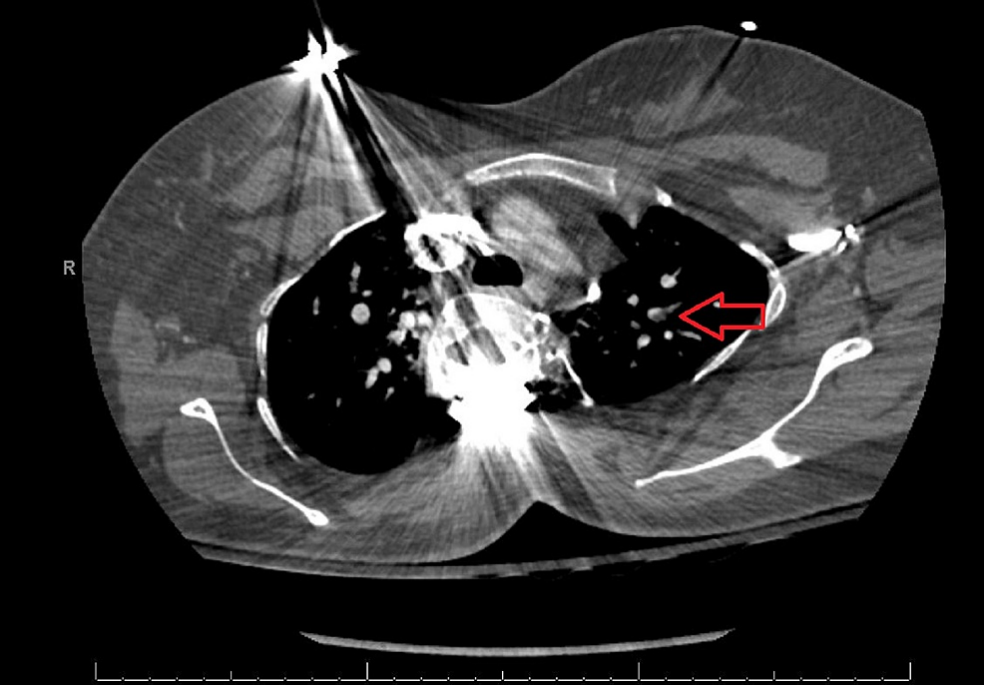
CT pulmonary angiography. Red arrow: evidence of a clot in the left upper subsegmental pulmonary artery branch. CT: computed tomography
